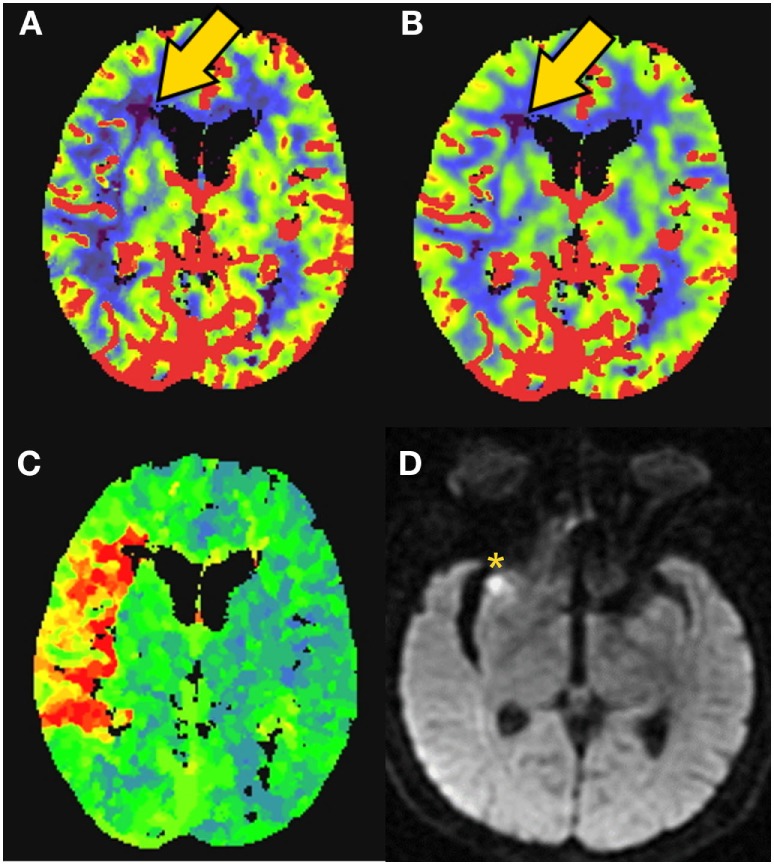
CT perfusion to identify salvageable tissue. 81-year-old female with right middle cerebral artery (MCA) syndrome and occlusion of the MCA on CT-angiogram. CT-perfusion maps: (A) cerebral blood flow (CBF), (B) cerebral blood volume (CBV), and (C) time to peak (TTP). Elevated time-to-peak contrast enhancement (TTP) colors orange to red represent >6-s delay (C). Severely low blood flow and volume territories are violet color (gold arrow). The patient was rapidly revascularized and the final infarction is demonstrated on MRI diffusion weighted imaging [(D)––yellow asterisk]. Data analysis for figure was created under approval of local ethics committee.
